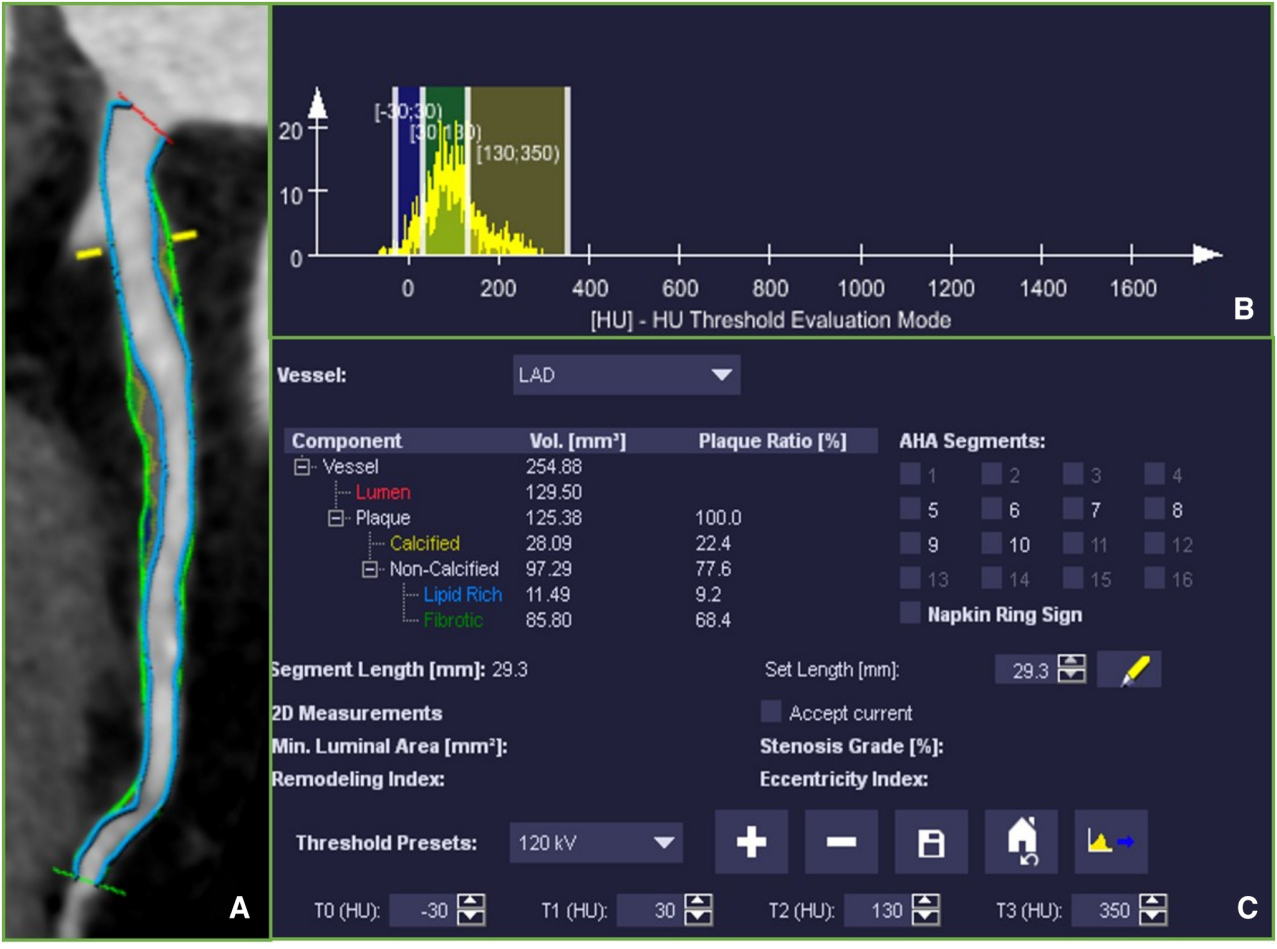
(A) Multiplanar reconstruction of the left anterior descending coronary artery (LAD) showing the whole coronary artery lesion, ranging from the proximal to distal lesion marker (green markers). Fibrotic tissue was labeled in dark yellow, Fibro-fatty tissue in light green and necrotic core was labeled in blue. (B) A histogram of the distribution of CT attenuation within the plaque. (C) Plaque volumes were derived for the quantitative analysis software based on pre-set thresholds.
